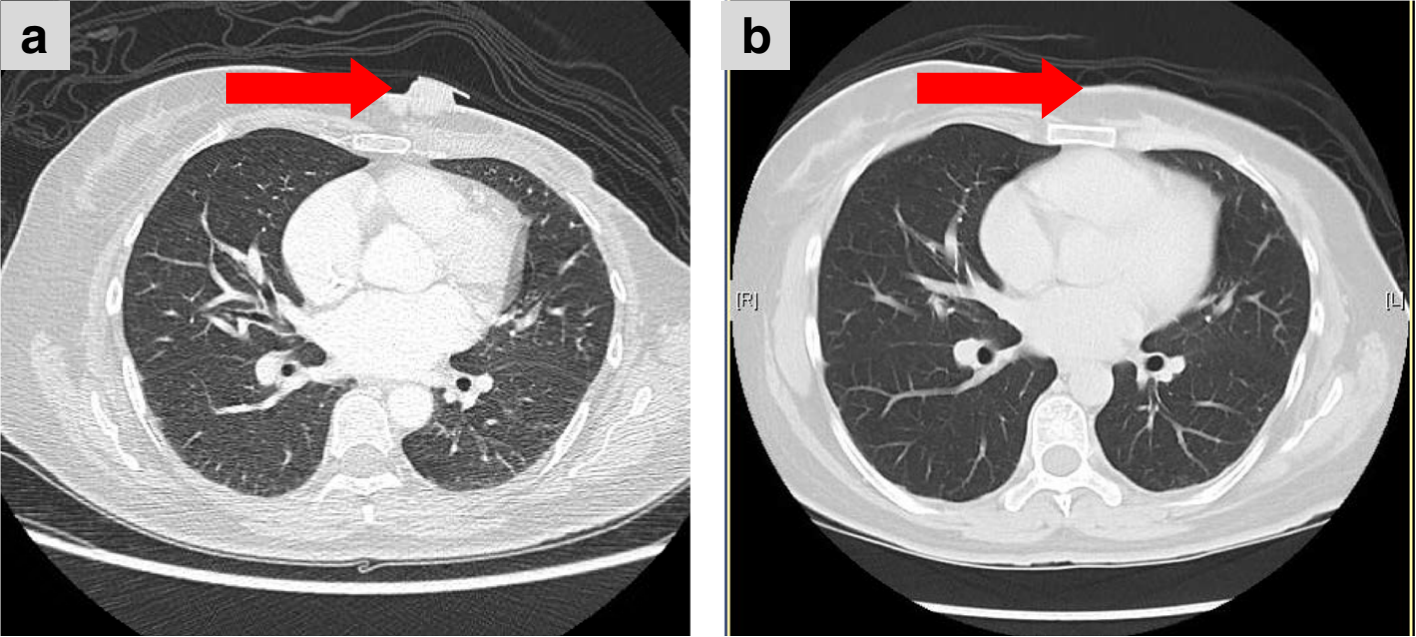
Baseline and follow-up CT scans. Baseline (a) and follow-up (b) CT scans of a 43-year-old patient with left chest wall soft tissue metastases
